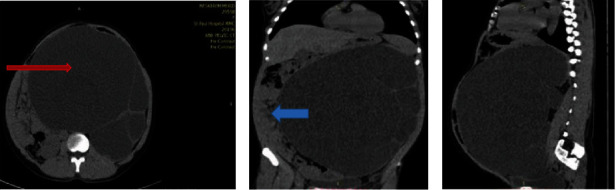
Noncontrast CT scan of the abdomen shows (a) axial view, (b) coronal view, and (c) sagittal view. It shows 20 cm long, 16 cm width cystic swelling (red arrow) with significant mass effect to the surrounding bowel loop (blue arrow).
